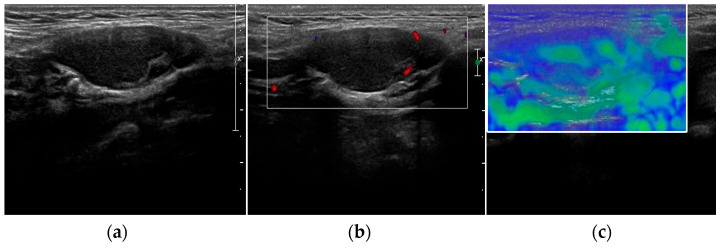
Grey-scale (a), Color Doppler (b) and elastography (c) aspect of a infected second branchial cleft cyst.
